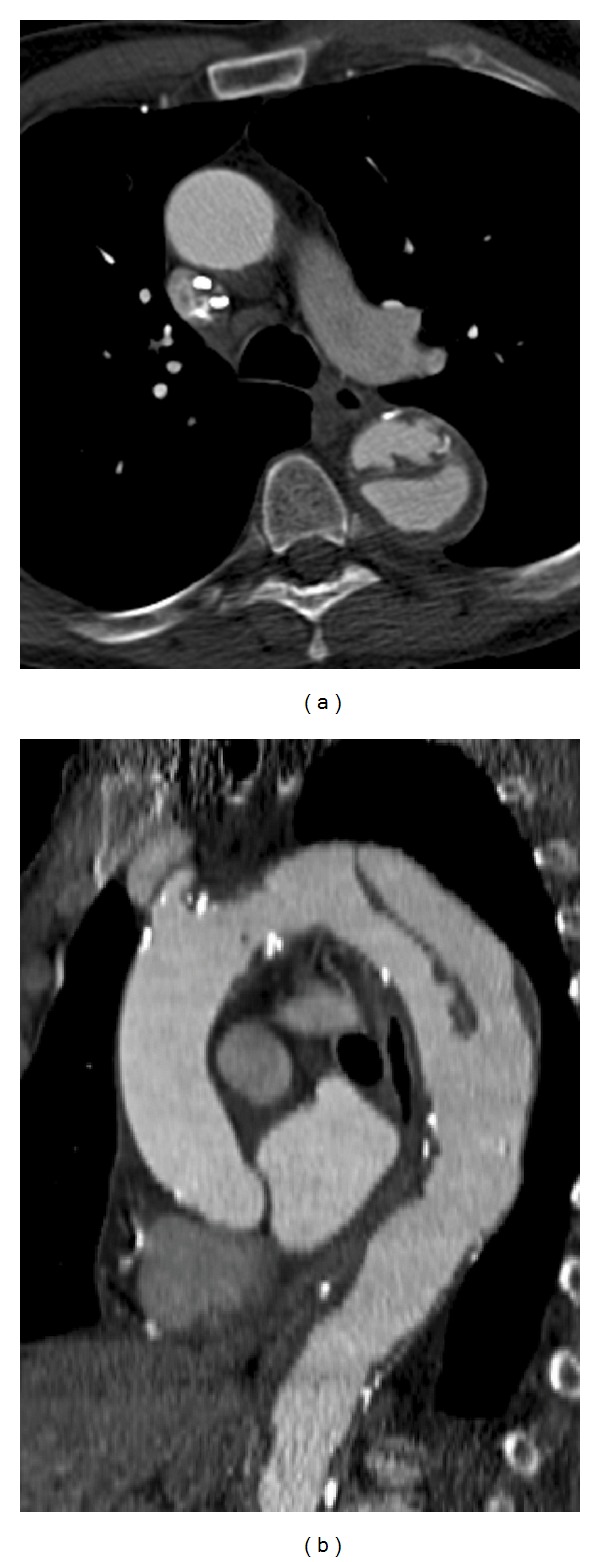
Type  B  dissection—(a) axial CT scan image in a 65-year-old male shows a dissection flap seen only in the descending thoracic aorta but not in the ascending aorta. (b) Sagittal reconstructed post contrast CT image in the same patient shows a dissection flap that involves the arch and proximal descending thoracic aorta, consistent with Type B dissection. The true lumen is anterior and medial, where the false lumen is posterior to lateral.
